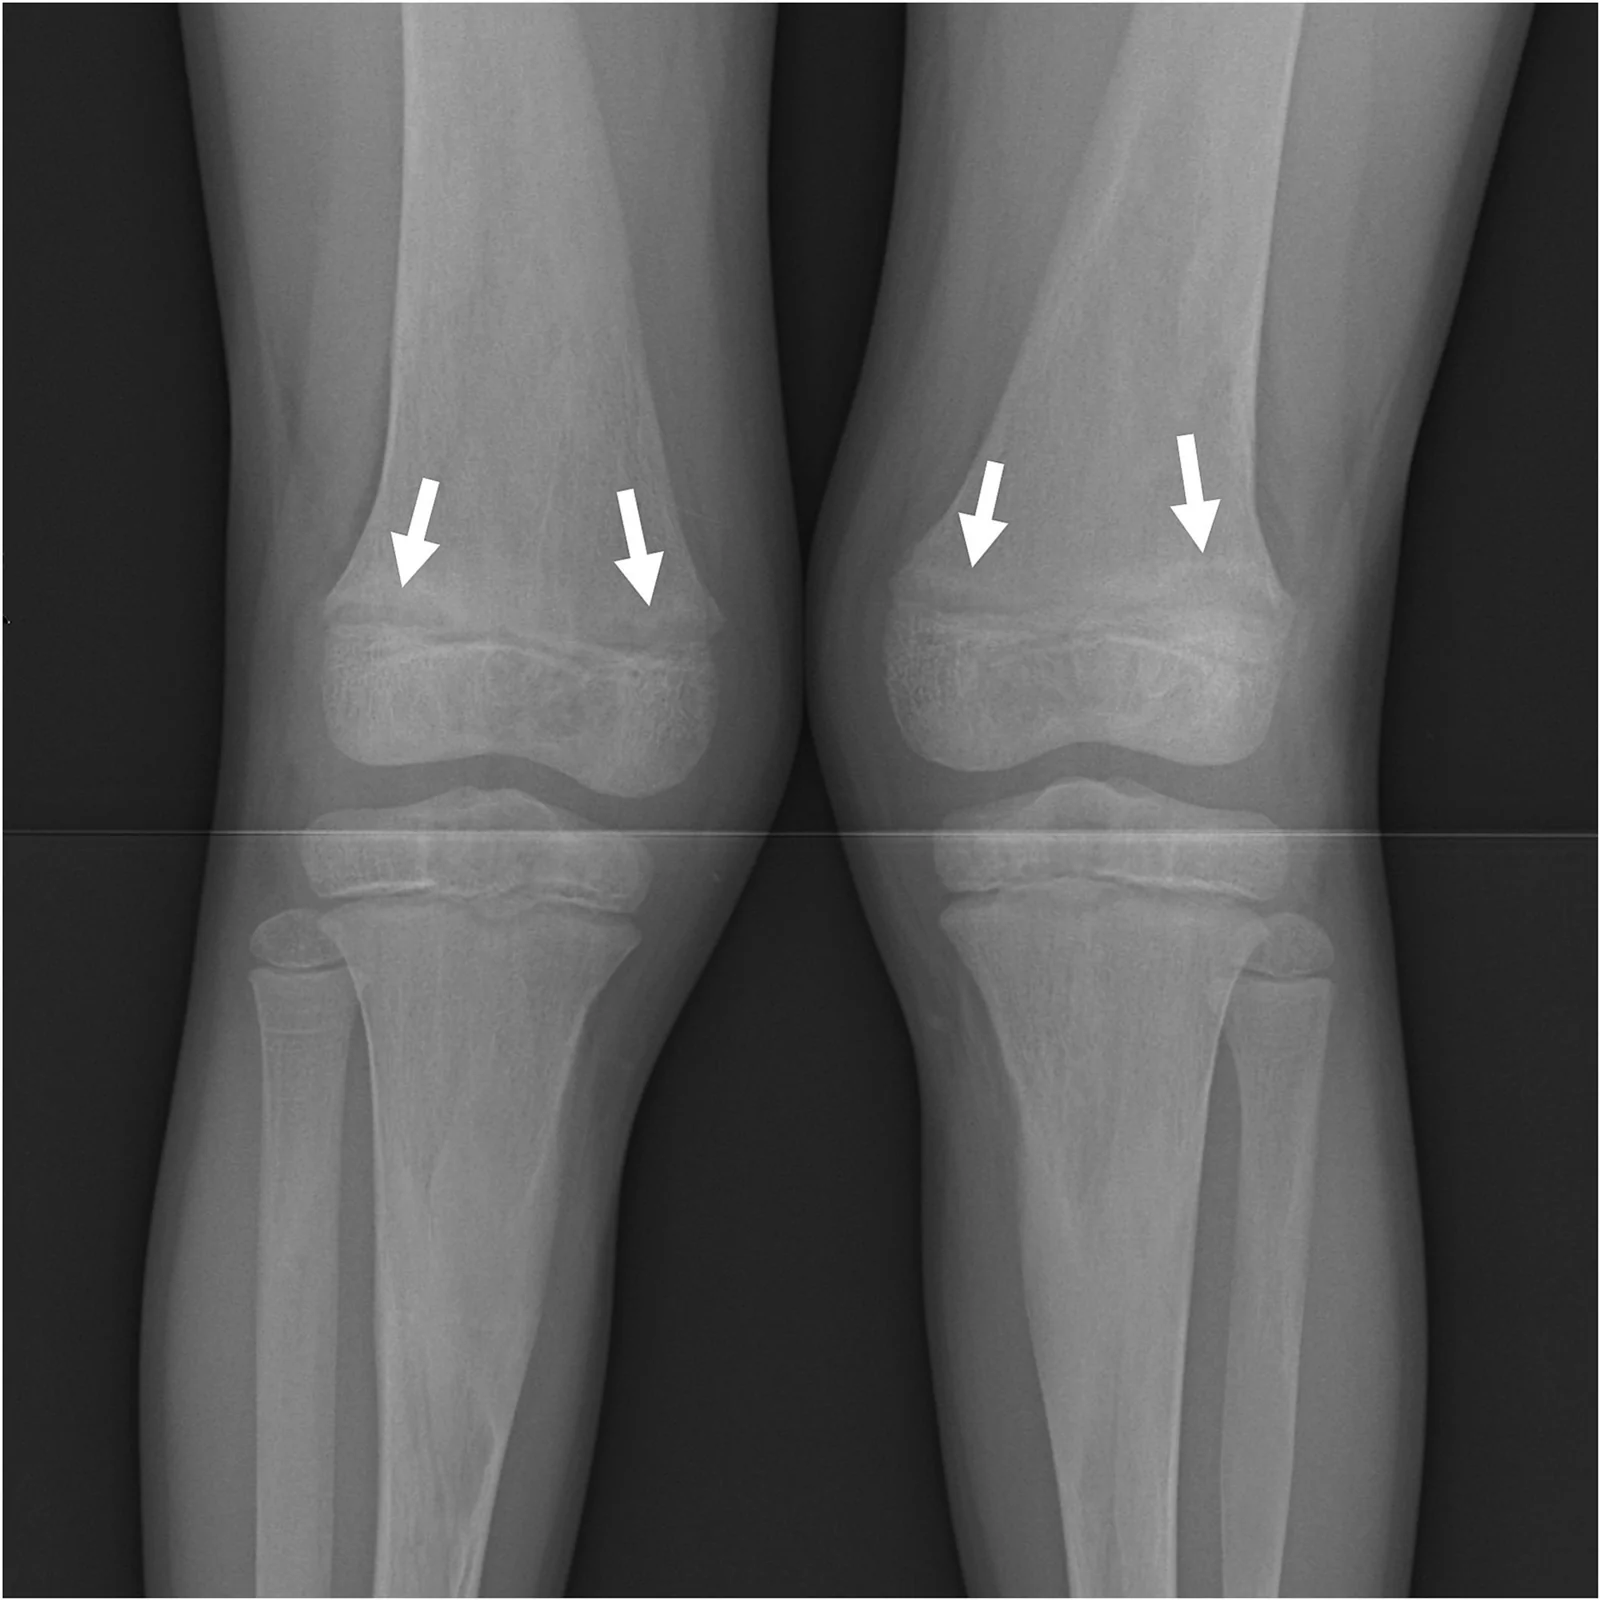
Previously unrecognized rachitic changes in Case 1 at age 8. Anteroposterior radiograph of bilateral knees showing distal femoral metaphyseal widening, flaring, and fraying with physeal irregularity. Arrows indicate metaphyseal irregularity adjacent to the distal femoral physes. These findings were retrospectively consistent with rachitic changes.
